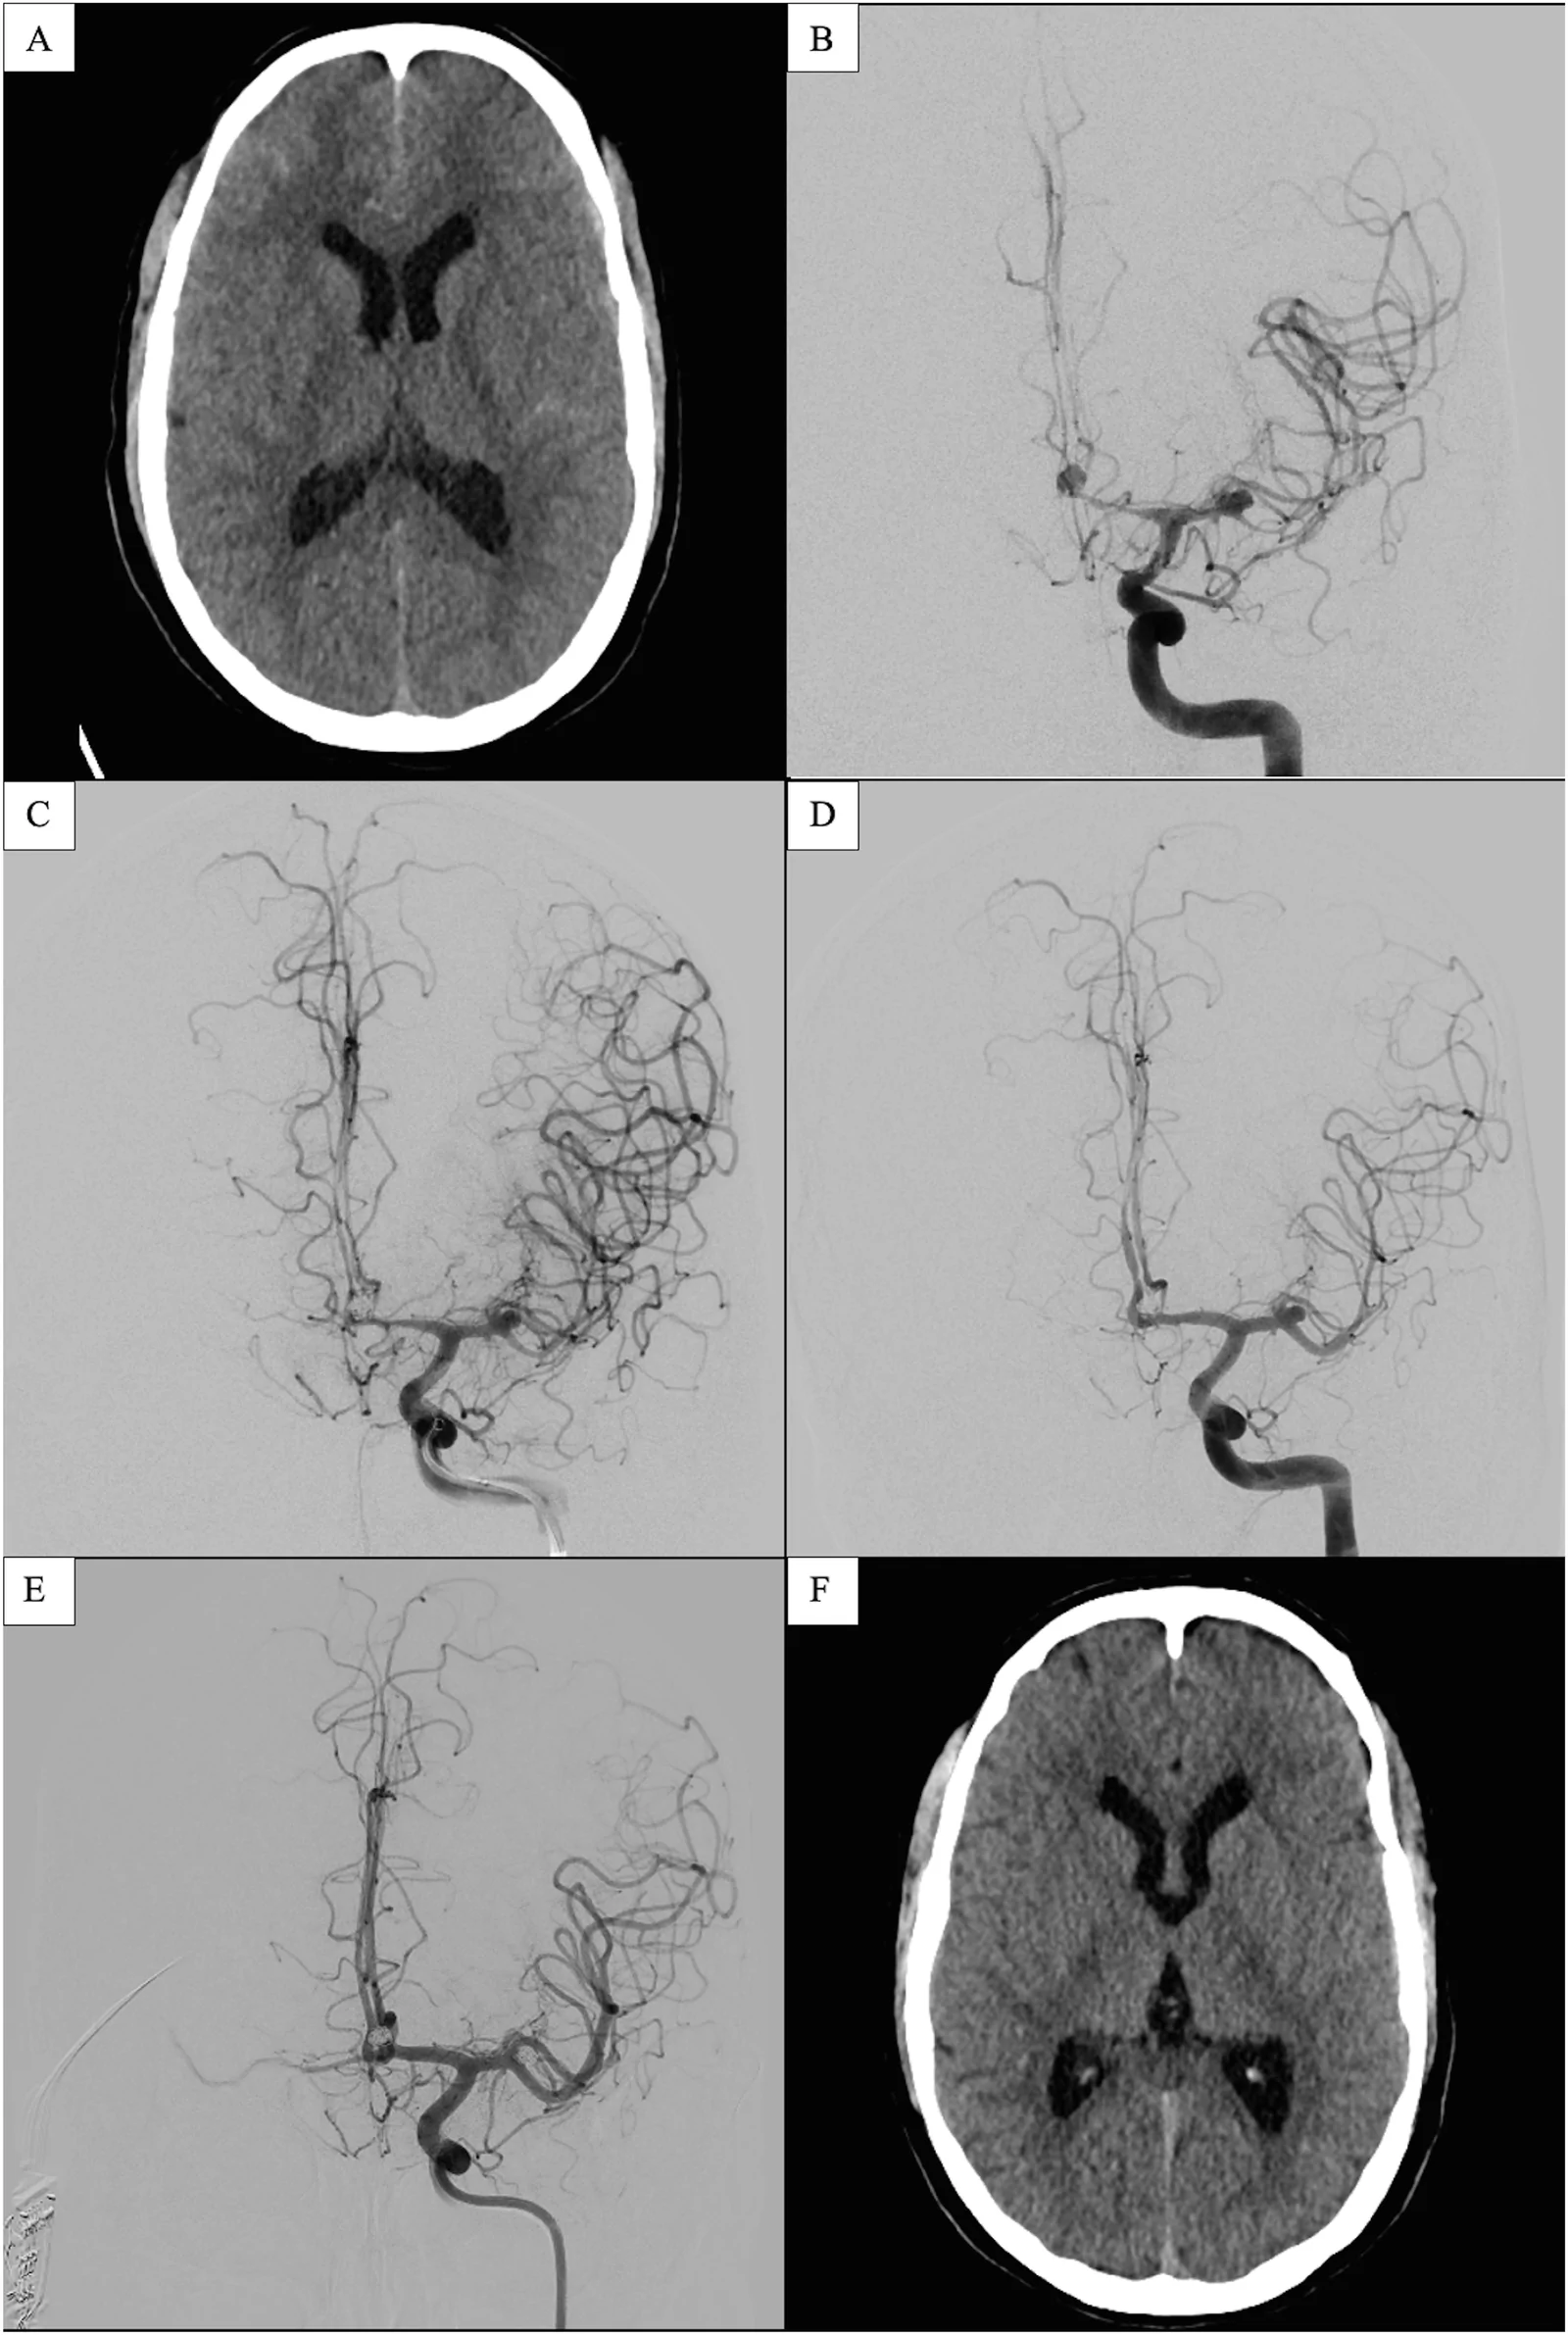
Computed tomography (CT) and digital subtraction angiography (DSA) images of a 44-year-old male, who had an acute onset of headache 9 days before the admission. His headache was initially clinically diagnosed as migraine, but he later presented to the emergency department due to acute-onset hemiparesis. 1A: A CT head scan with hyperdensity in cortical sulci suggesting subarachnoid hemorrhage. 1B: In the immediate DSA, intracranial aneurysms in the anterior communicating artery (ACoA) and left middle cerebral artery bifurcation were found concurrently with severe vasospasm of both anterior and middle cerebral arteries. 1C: The ACoA aneurysm was considered ruptured, and it was endovascularly coiled immediately after the diagnostic DSA. Intra-arterial nimodipine (IAN) was administered during the treatment and the vasospasm slightly decreased at the end of the procedure. IAN was administered during three consecutive days. Mechanical angioplasty was also conducted due to the patient's serious symptoms and severe vasospasm. 1D: After the third IAN administration, the spasm had significantly decreased. 1E: The MCA aneurysm was coiled later and vasospasm is no longer visible. 1F: No ischemic lesions were found on the post-treatment CT scan. Clinically, the patient made an excellent recovery with a 6-month mRS of 0.
